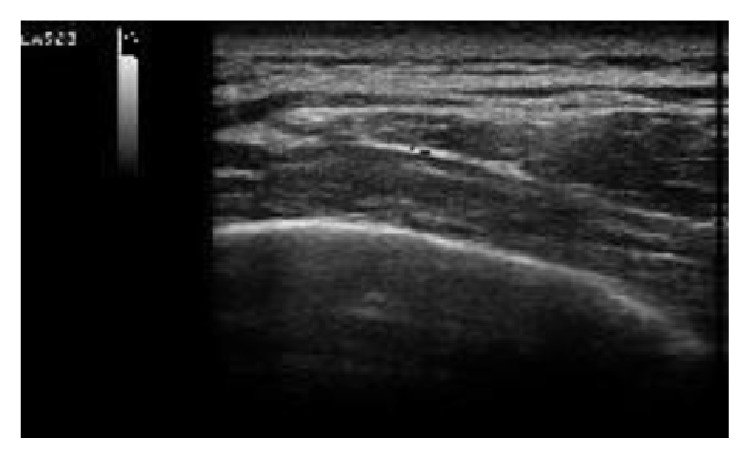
Ultrasound (US) echographic of right lung made in September 2014 after treatment. Disappearance of Ultrasound Lung Comets (ULCs) at the fifth right posterior intercostal space.
